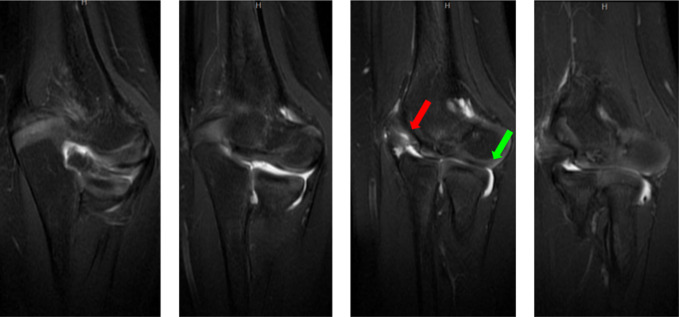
Radiographs demonstrating the sucessive coronal T2-weighted MRI images of the left elbow. Breakdown of normal cartilaginous surface of the trochlea shown (red arrow). For comparison, the normal cartilage of the capitellum is also demonstrated (green arrow).
